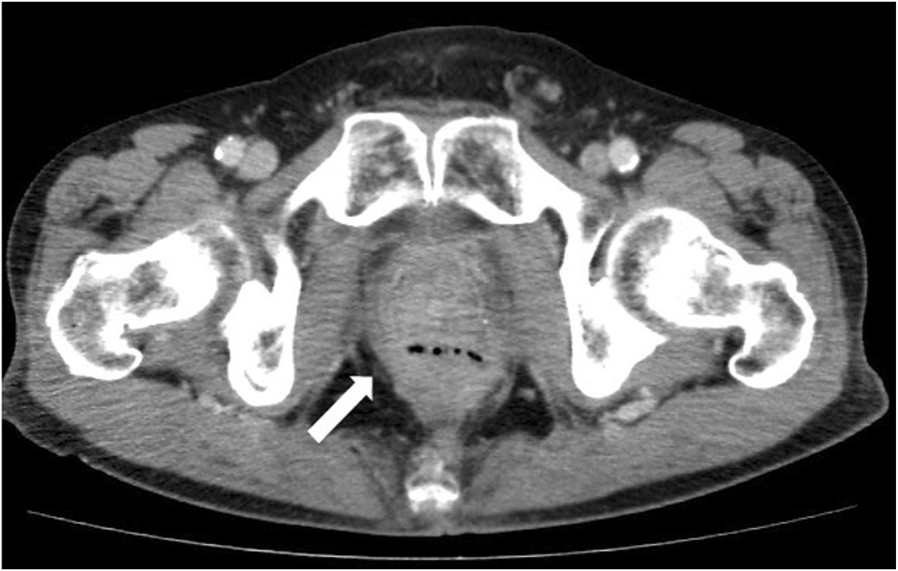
Computed tomography scan of the abdomen revealed a small amount of extraluminal air between the prostate and rectum, adjacent to the anastomotic site (white arrow)
